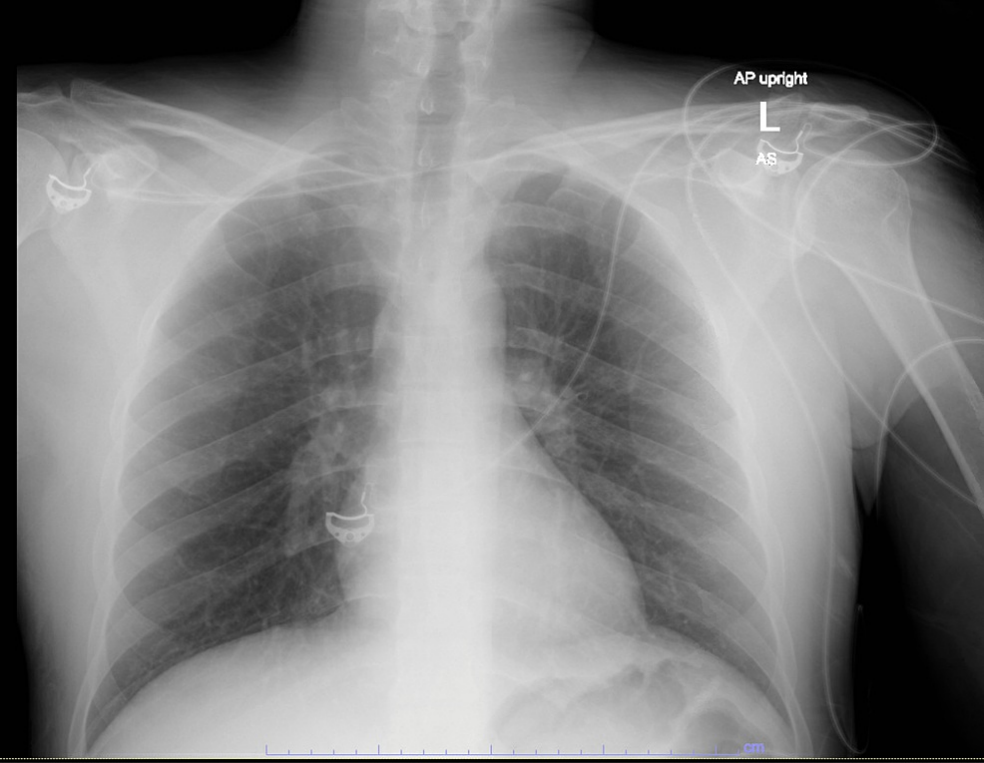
AP chest X-ray from admission
